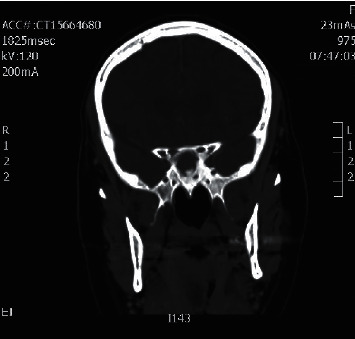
Coronal CT scan sinuses showing a sphenoid opacity of fungal ball post endonasal endoscopic pituitary surgery.
